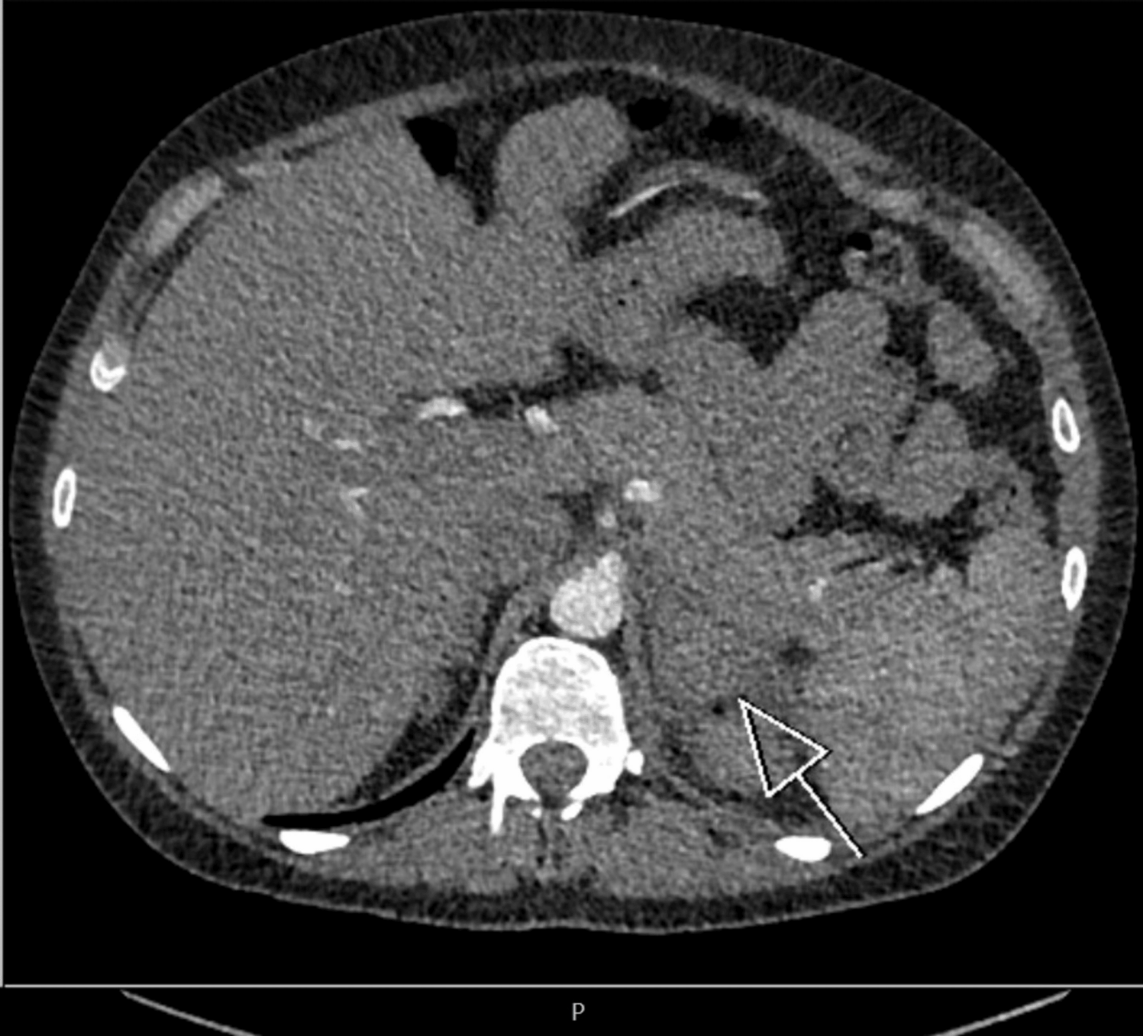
Axial CT pulmonary angiogram showing an enlarged left adrenal gland (arrow).
